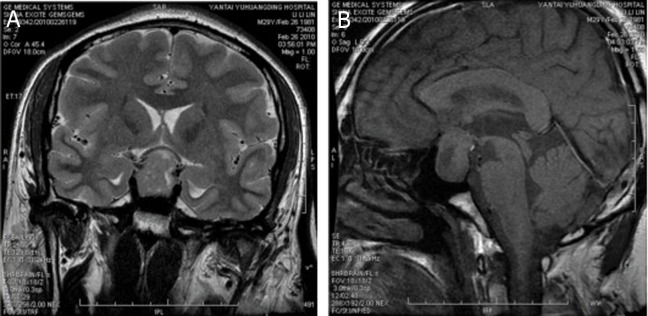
Cornal (A) and sagittal (B) MRI sections revealed ahuge well-enhanced mass in the sella turcica.
